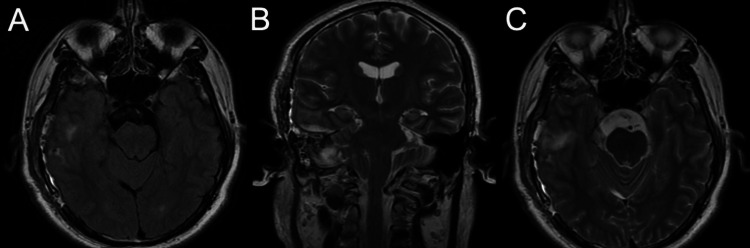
Postoperative MRI. Follow-up MRI at three months post-surgery showing a fully decompressed mesial temporal lobe and a minimal residual of the capsule in an axial FLAIR image (A), coronal T2-weighted image (B), and axial T2-weighted image (C).
